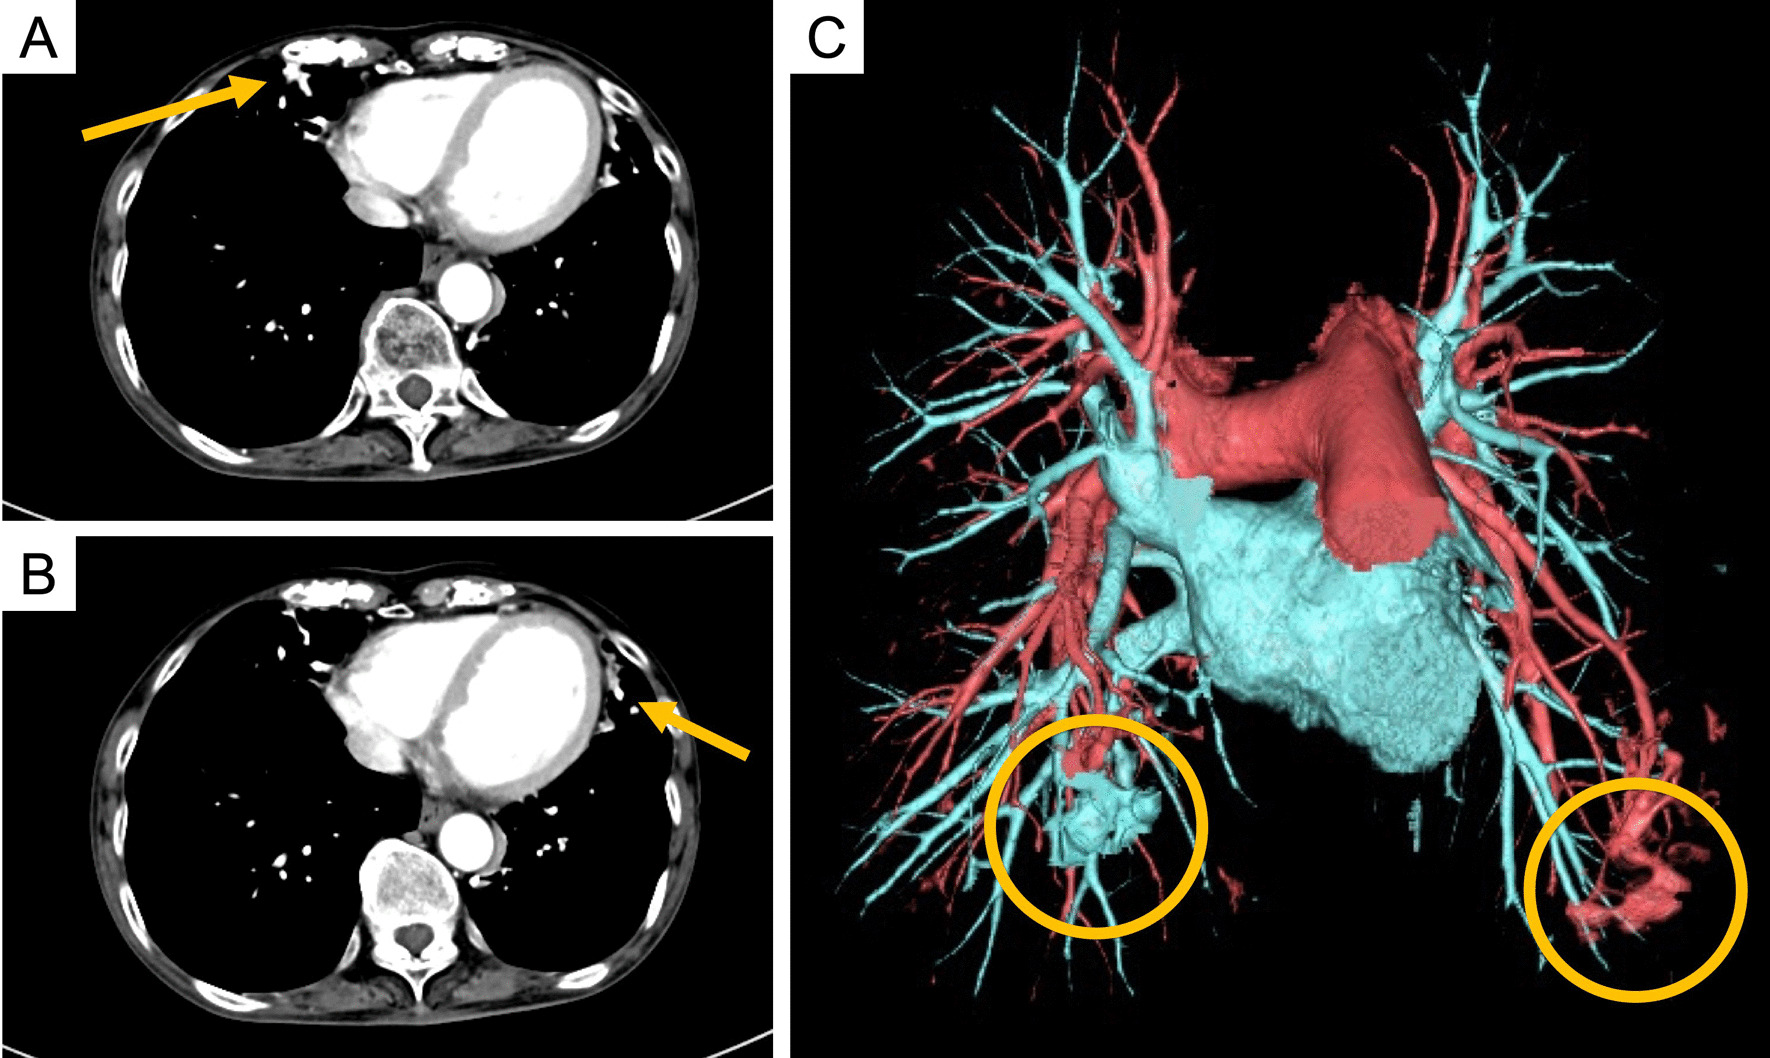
Findings of contrast-enhanced chest computed tomography and reconstructed 3D-contrast-enhanced computed tomography images. Contrast-enhanced chest computed tomography images show pulmonary veins of right middle lobe and left lingular segment in arterial phase (A, B; arrow). Reconstructed 3D-contrast-enhanced computed tomography image shows abnormal intrapulmonary shunts in right and left pulmonary artery and vein (C; circles)
